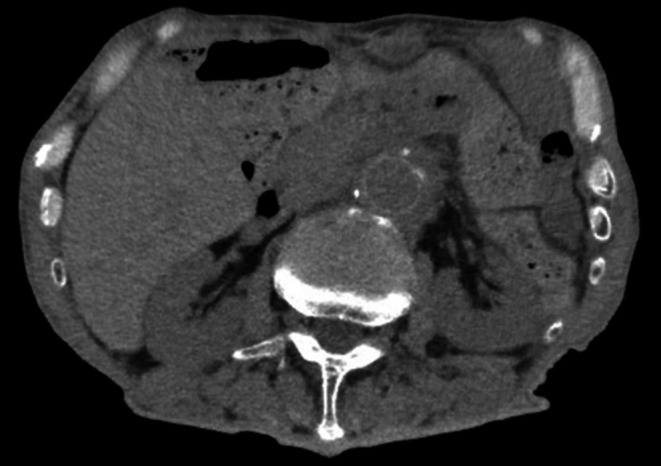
Postoperative computed tomography. Follow‐up CT 6 months after prosthetic graft replacement demonstrates complete resolution of the periaortic inflammation and no evidence of aneurysmal recurrence.
